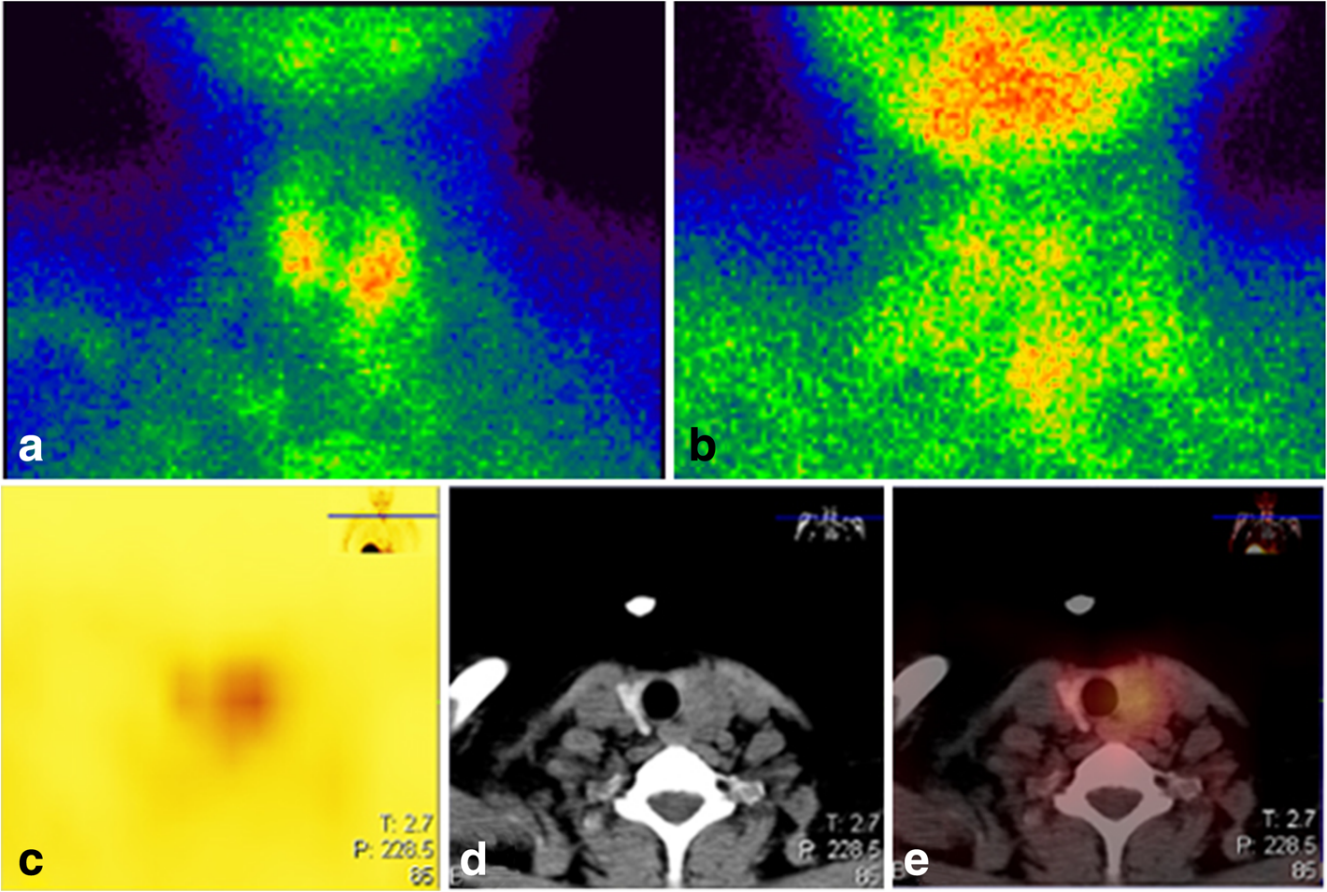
Representative ^99m^Tc-MIBI thyroid images indicative of hyperparathyroidism. The patient was intravenously injected with 740–925 MBq^99m^Tc-MIBI. A single-photon emission (SPECT)/CT instrument was used, which was equipped with a parallel low-energy high-resolution collimator with an energy peak of 140 keV and a window width of 20%. The magnification when performing the neck scan was 1.5-fold, with a matrix of 128 × 128 pixels and an acquisition count of 1000 K at 10 min and an acquisition count of 682 K at 120 min ^99m^Tc-MIBI injection. Two points have the same acquisition time of 600 s. Neck systemic planar images were obtained after 10 min a and 120 min b; c-e There is a soft tissue nodule located in the posterior lobe of the left thyroid gland, with radioactive accumulation
